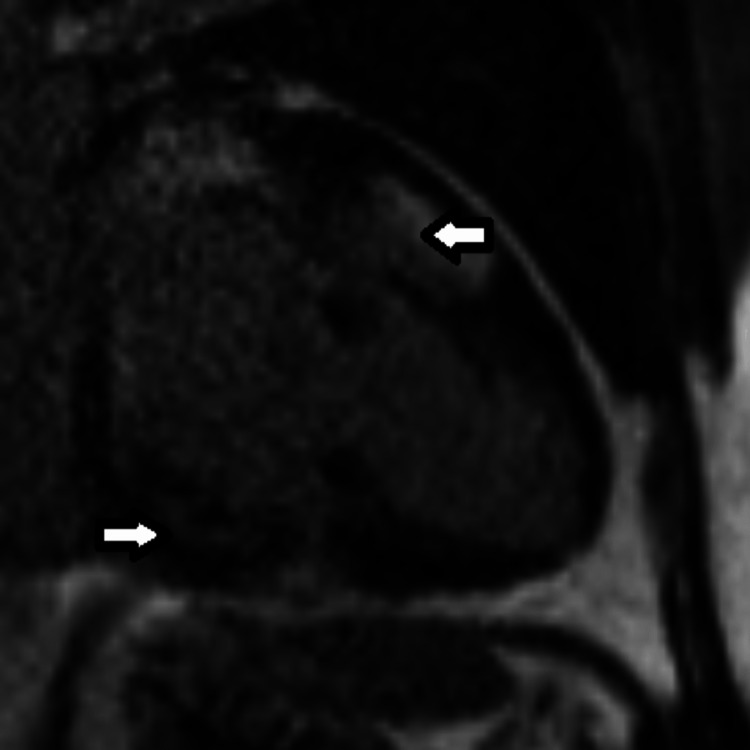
Cardiac MRI (Two-chamber view). Extensive areas of pathologic late gadolinium enhancement with mid myocardial and epicardial pattern with near complete transmural involvement, consistent with infiltrative heart disease (arrows).
